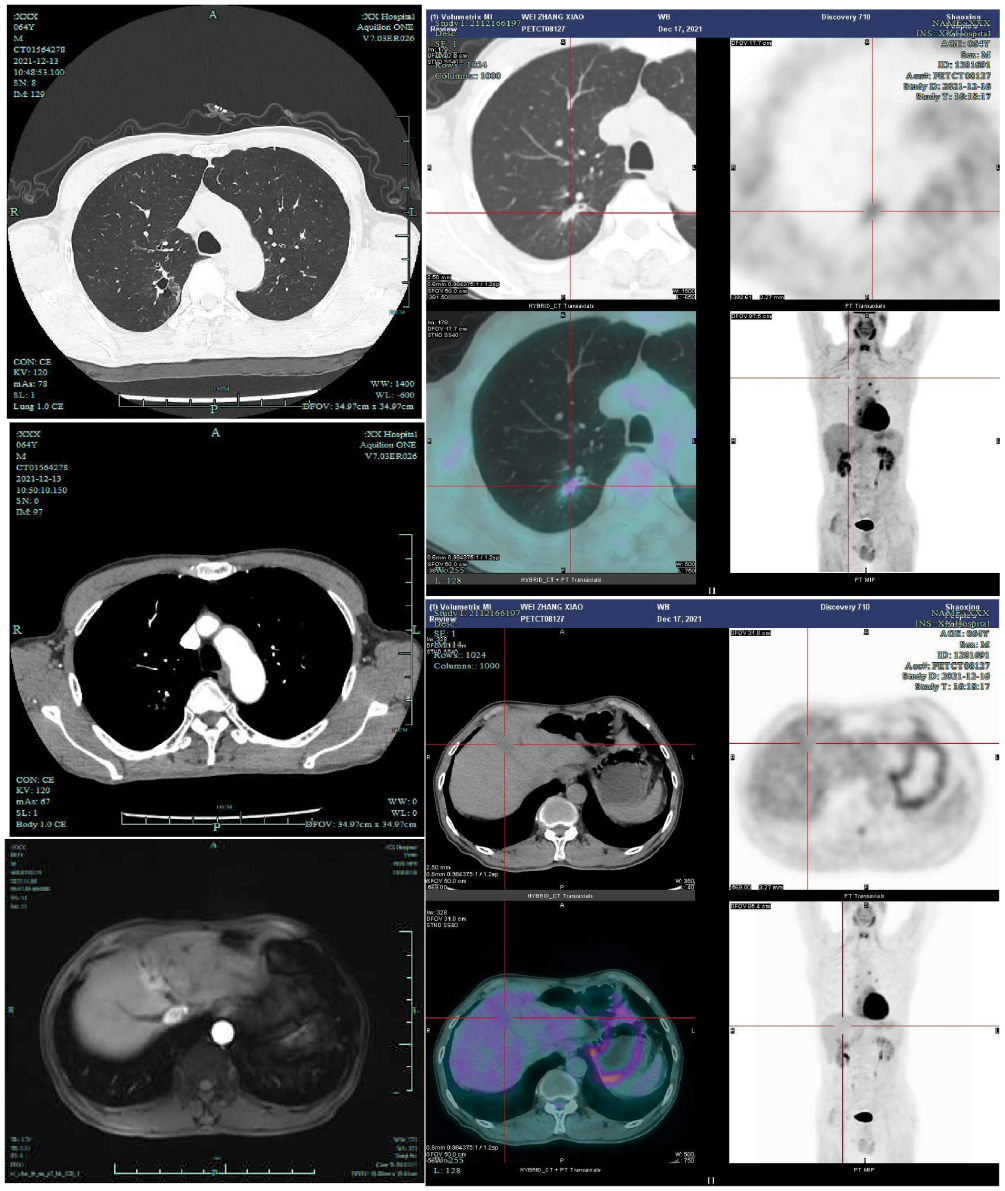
Preoperatively, the patient underwent contrast-enhanced chest CT (2021-12-13), contrast-enhanced liver MRI (2021-11-9), and PET-CT examinations (2021-12-17). Preoperative PET-CT demonstrated a right upper lung nodule with a maximum standardized uptake value (SUVmax) of 1.85, while the liver mass exhibited physiological FDG uptake.
